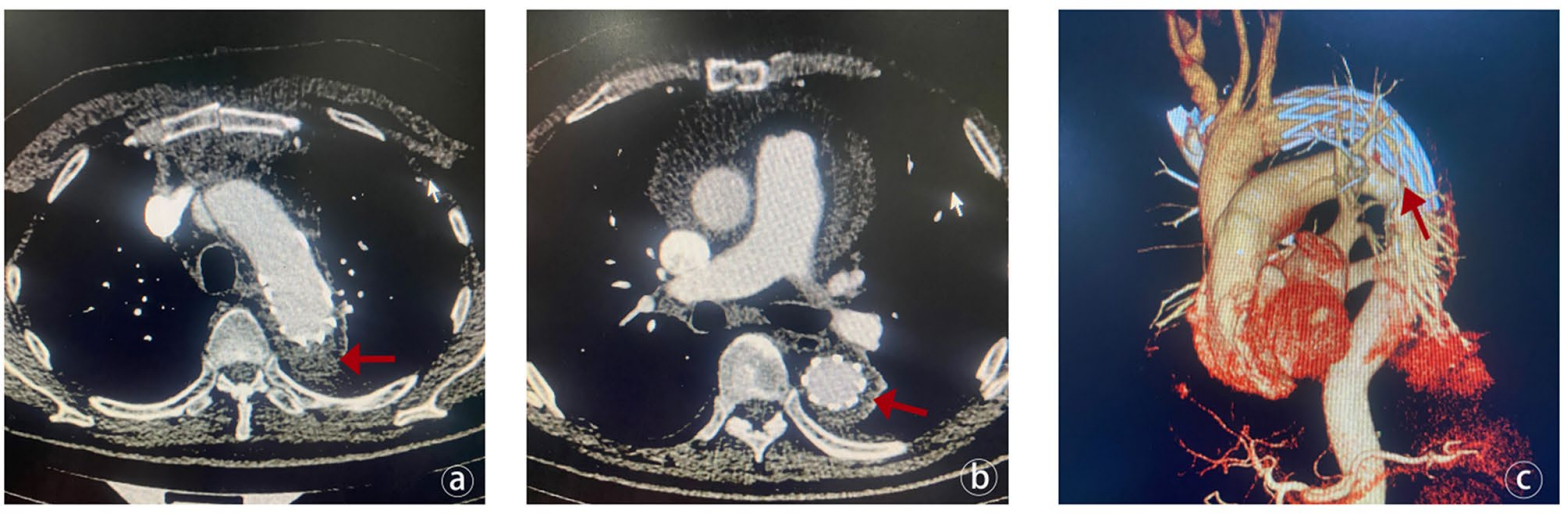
Assessment of stent and thrombus status after TAAR. (a) The red arrow indicates that the thrombus in the false lumen of the aortic arch has been thrombosed after surgery, and the stent is in good shape. (b) The yellow arrow indicates that the thrombus in the false lumen of the descending aortic stent has been completely thrombosed after surgery, the stent is in good shape, and there is no endoleak. (c) The 3D reconstruction of the patient’s aortic CTA demonstrates the morphology of the stent
